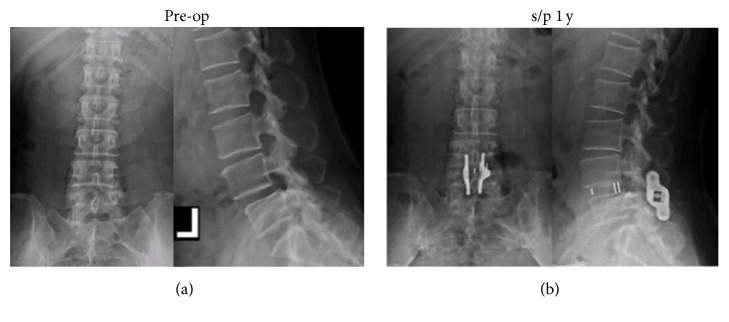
Imaging studies obtained in a 59-year-old female who presented with grade I spondylolisthesis (a). The patient underwent interspinous fusion device implantation following PLIF. One year after surgery, anteroposterior and lateral radiographs confirmed the position of the IFD and cage and showed both interbody and interspinous bone fusion (b).
